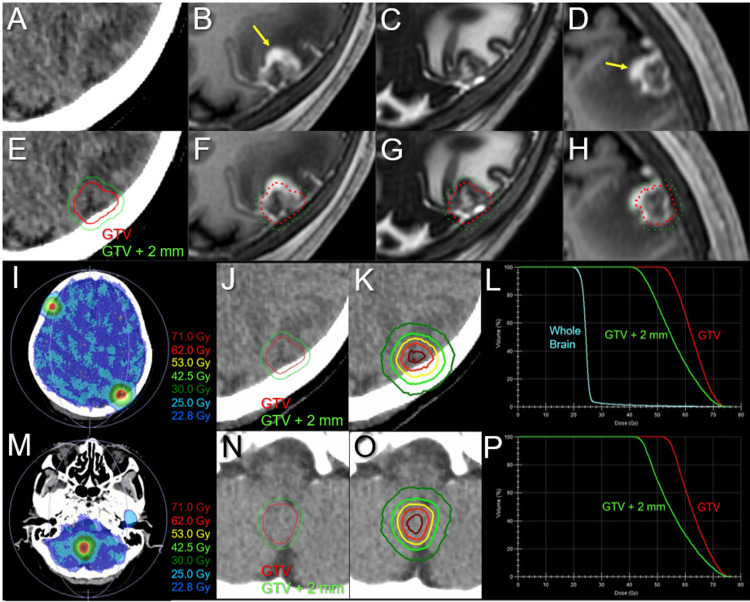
Target definitions, dose distributions, and dose-volume histograms for the third 10-fr stereotactic radiosurgery combined with simultaneous whole-brain irradiation. The images show (A, E) non-CE-CT images and (B, D, F, H) CE-T1-WIs, (C, G) T2-WIs, (I, K, M, O) dose distributions, (E-H, J, N) target definitions, (L, P) DVHs, (A-C, E-G, I-K, M-O) axial images, (D, H) sagittal images, (A-L) the left parietal lesion with local progression following prior SRS, and (M-P) the new right cerebellar lesion. (A-H) These images are shown at the same magnification and coordinates under co-registration and fusions. (A-H) The GTV was defined, mainly based on the visible mass on the T2-WIs (C, G), with the exclusion of excessive exudation of contrast media (arrows in B and D) by contrasting CE-T1-WIs and T2-WIs (T1/T2 matching). (E, J, N) The GTVs and isotropic 2-mm margin-added structures. (I, K, M, O) The representative isofill doses (I, M) and IDLs (K, O) superimposed onto non-CE-CT images. (I, L, M) *D*_98%_ and *D*_50%_ of the whole brain are 22.8 and 24.5 Gy, respectively. CE, contrast-enhanced; CT, computed tomography; WIs, weighted images; DVHs, dose-volume histograms; SRS, stereotactic radiosurgery; GTV, gross tumor volume; IDLs, isodose lines; *D*_*X*%_, a minimum dose encompassing at least *X*% of the target volume; fr, fraction
